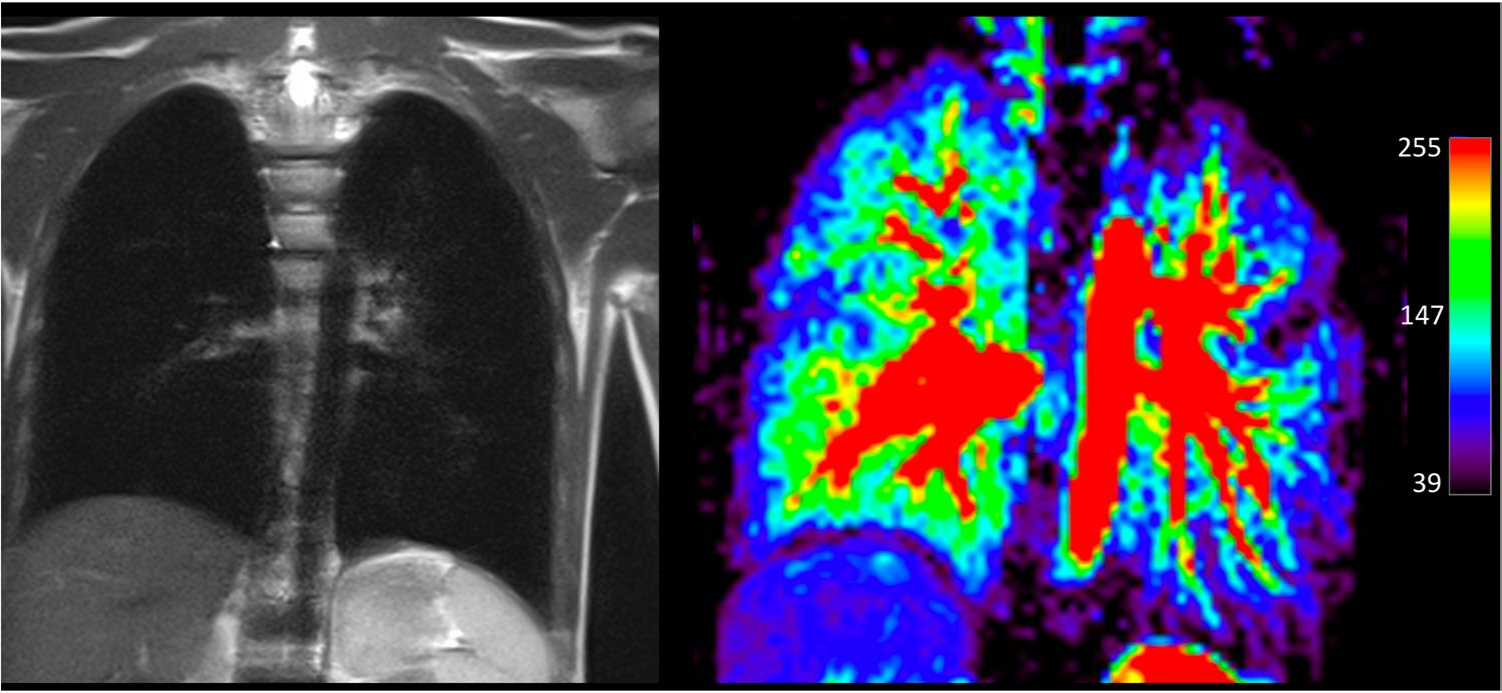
Ten-year-old patient after CDH repair on the left side. Left: Coronal T2-weighted image. Right: Pulmonary blood flow map. Already visually, reduced perfusion is recognized on the ipsilateral side. Color scale on the right codes perfusion values (mL/100 mL/min)
